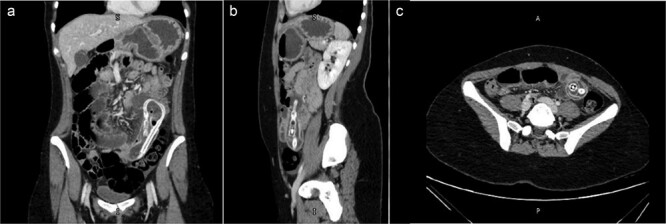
(a) Coronal view (b) sagittal view (c) axial view of the abdominal CT scan with oral water-soluble contrast showing IGB migrated and impacted in the jejunum with the proximal SBO.
